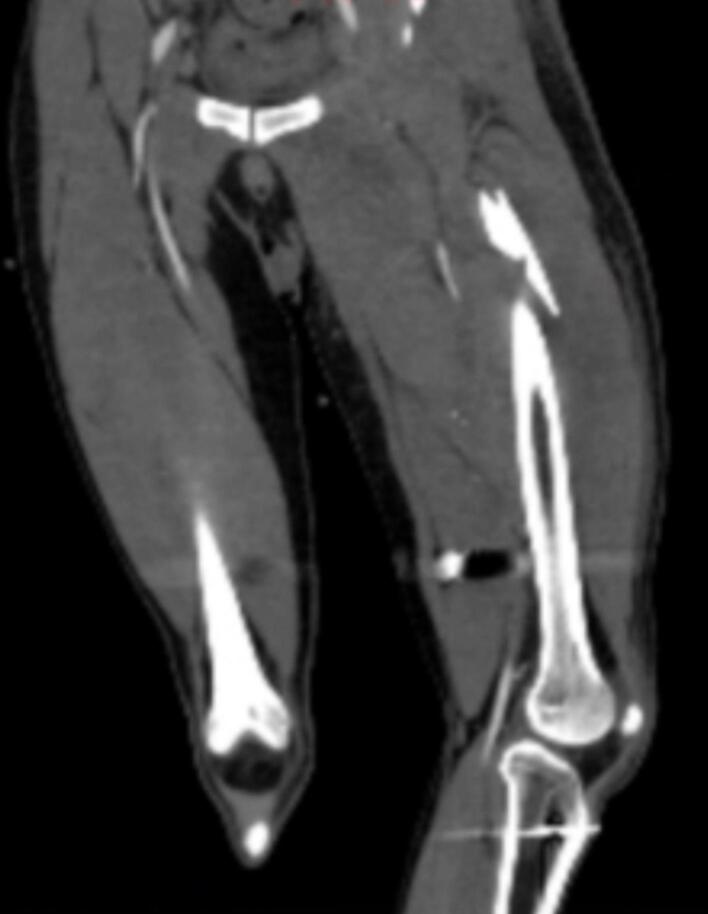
First-day computed tomography. Initial imaging indicated a left femoral bone shaft fracture without any evidence of pseudoaneurysm or fistula.
